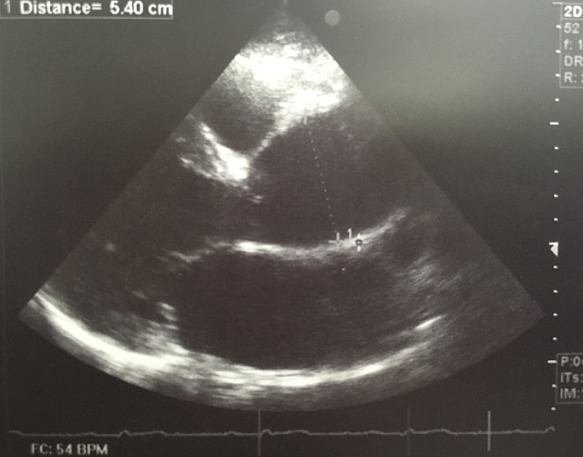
Echocardiographie trans-thoracique montre un anévrysme de l’aorte ascendante de 54 mm
